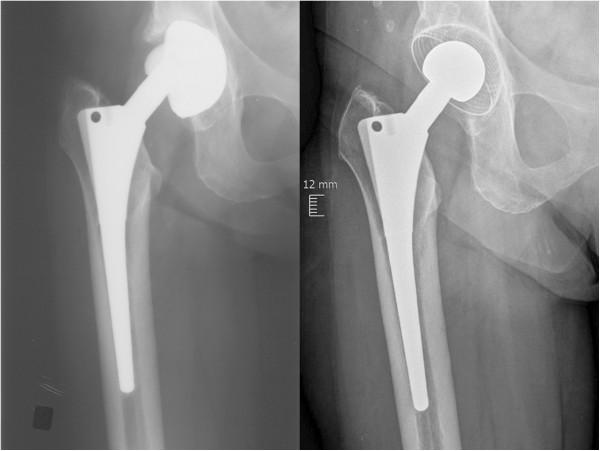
Representative X-rays taken before revision surgery and at 2 years post-surgery. The X-ray at left was obtained before revision surgery, and the one at right was taken at 2-year follow-up after revision surgery with a ceramys® revision head combined with an RM Classic Cup and a GSS-CO stem (Mathys Ltd Bettlach, Bettlach, Switzerland).
